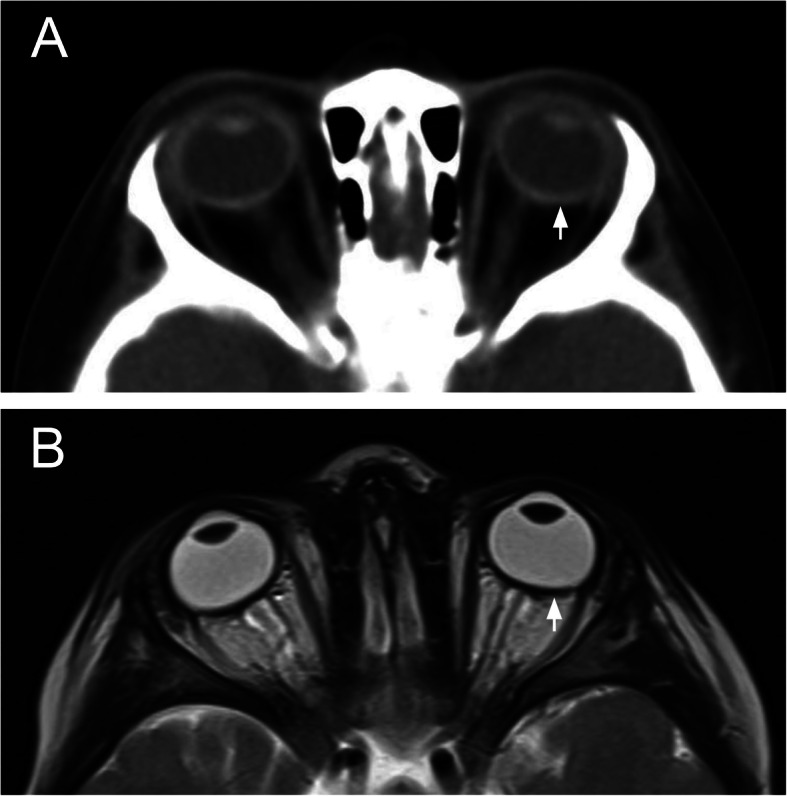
CT and MR imaging of patient (Nan3) with Thr518Met variant in *MYRF*. Axial CT (a) and axial T2 MRI (b) scans demonstrate short axial eye length and scleral appearance consistent with ultrasound measures and a diagnosis of nanophthalmos. A white arrow indicates sclera which is hyperdense in the CT image (a) and hypointense in the MR image (b)
